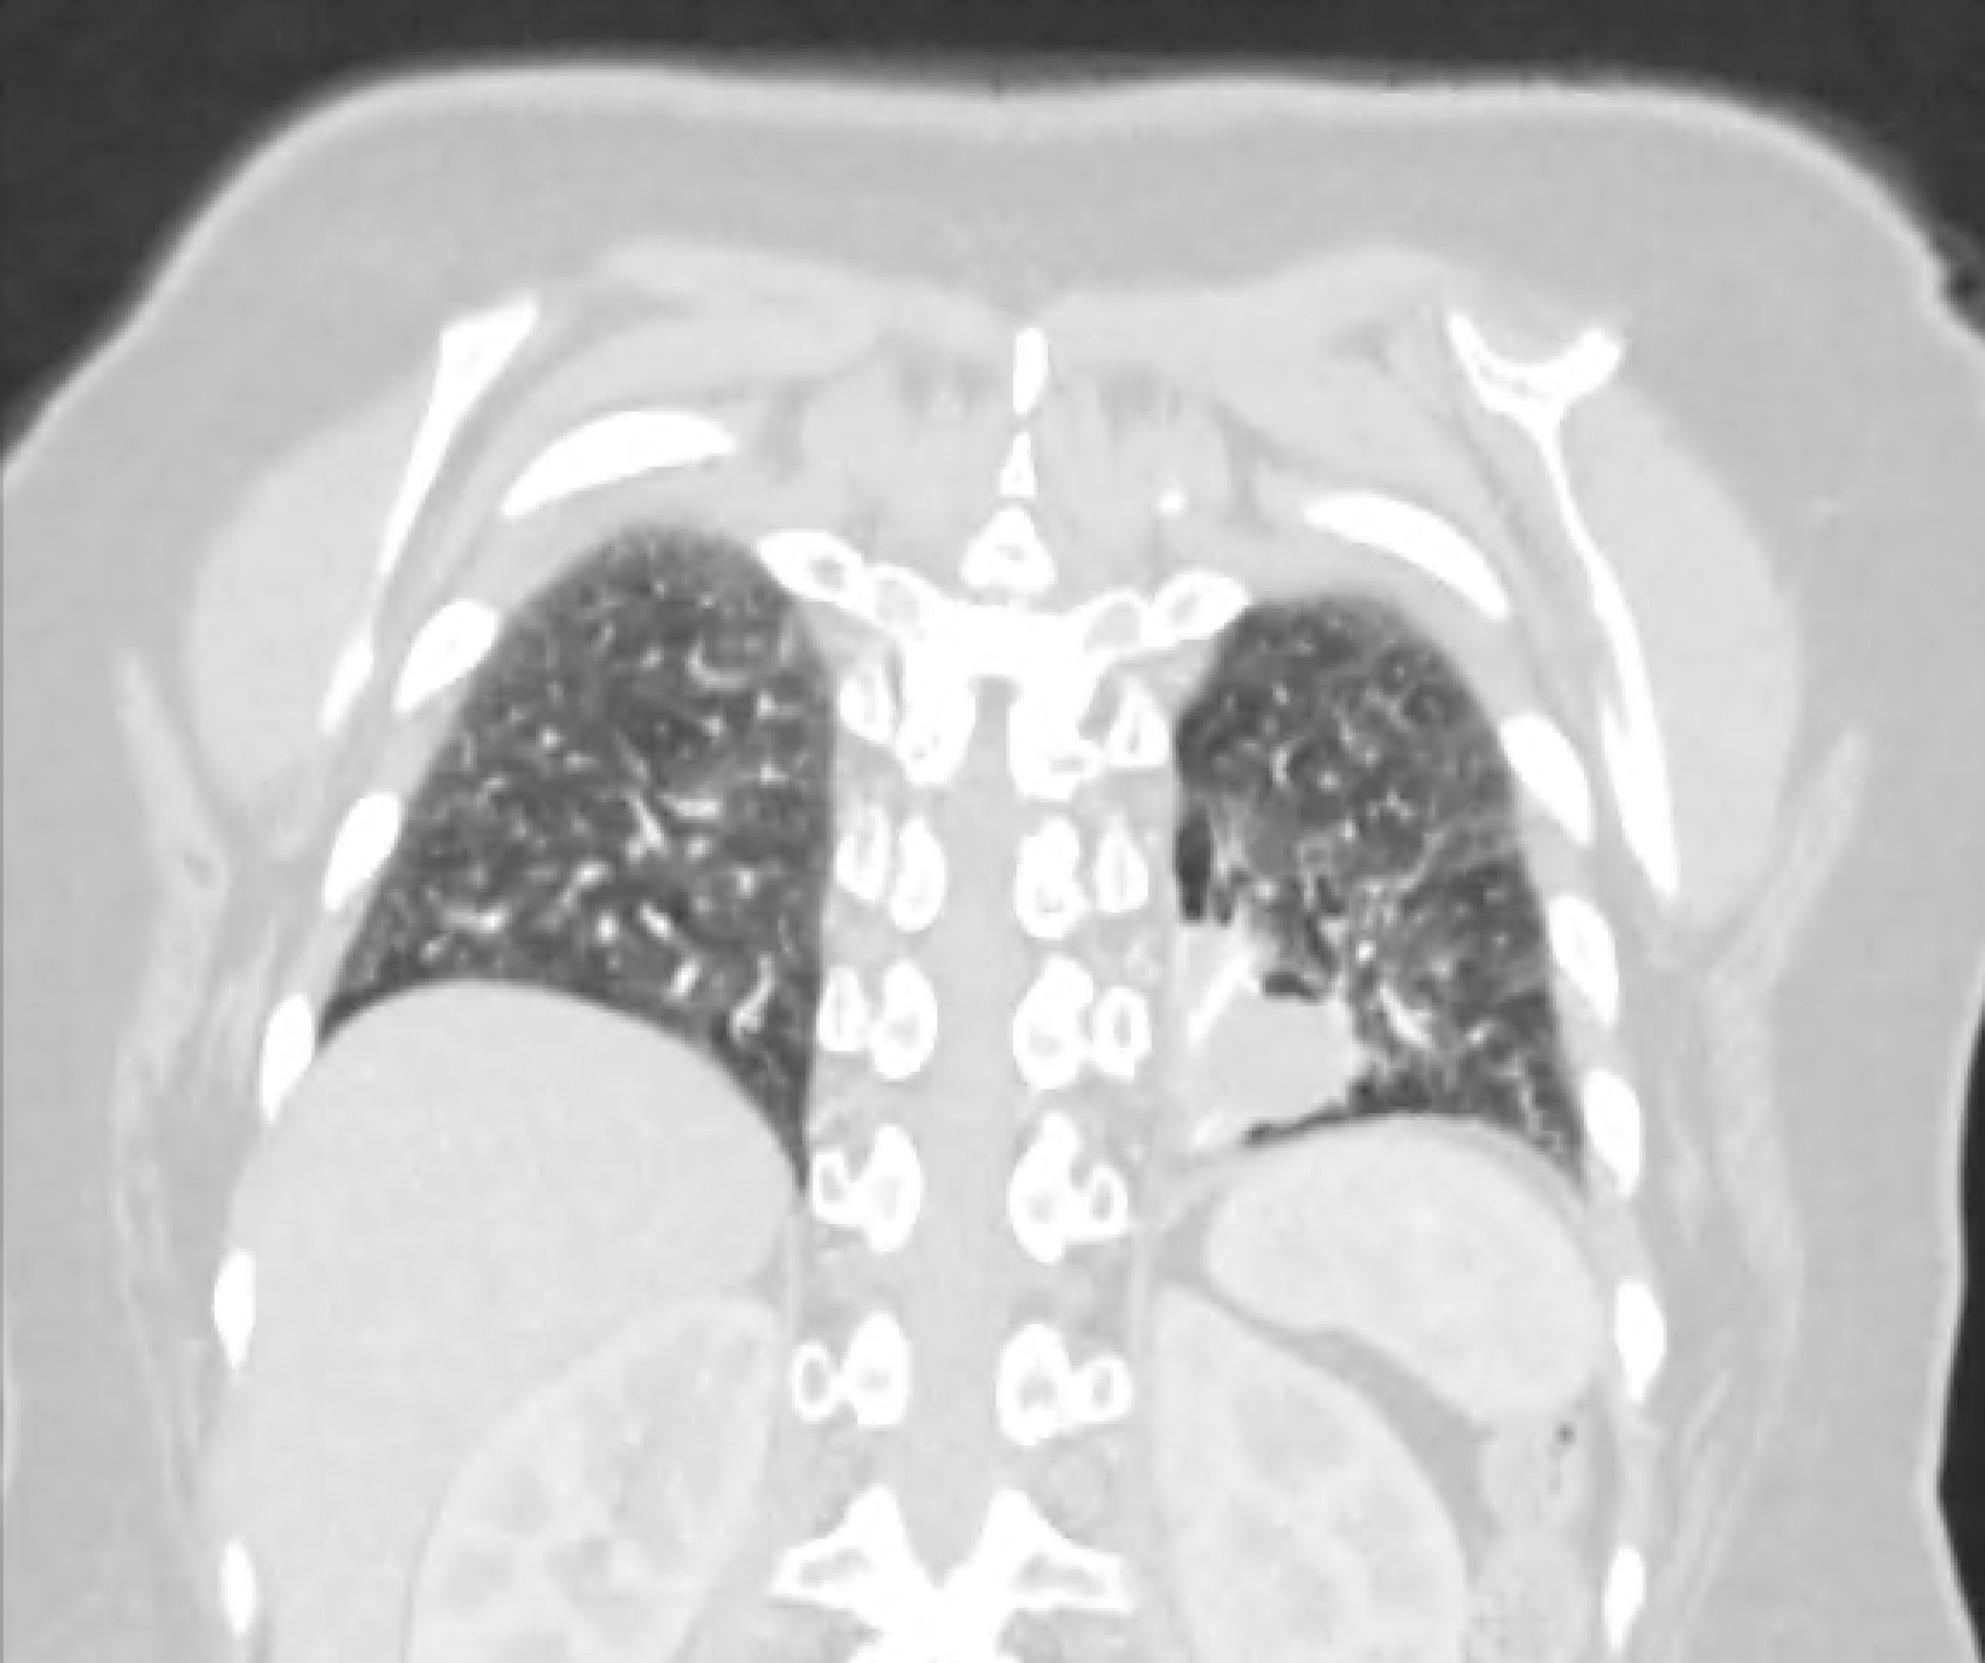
CT Chest coronal view showing left basal lung mass close to the spine
